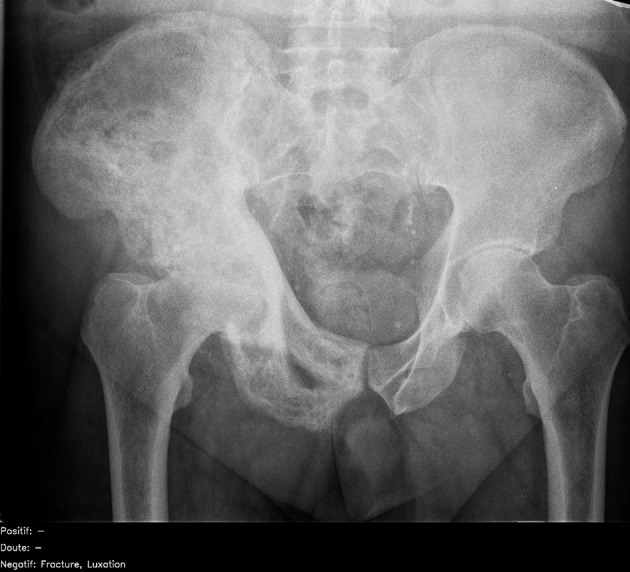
Abnormal adult pelvic radiograph showing increased sclerosis and expansion of right iliac bone in keeping with Paget’s disease. This was correctly identified by almost all radiologists (96%) but interpreted as normal by artificial intelligence candidate (false negative), given that this was not a pathology it was trained to identify. French translation: doute=doubt; luxation=dislocation; negatif=negative; positif=positive
